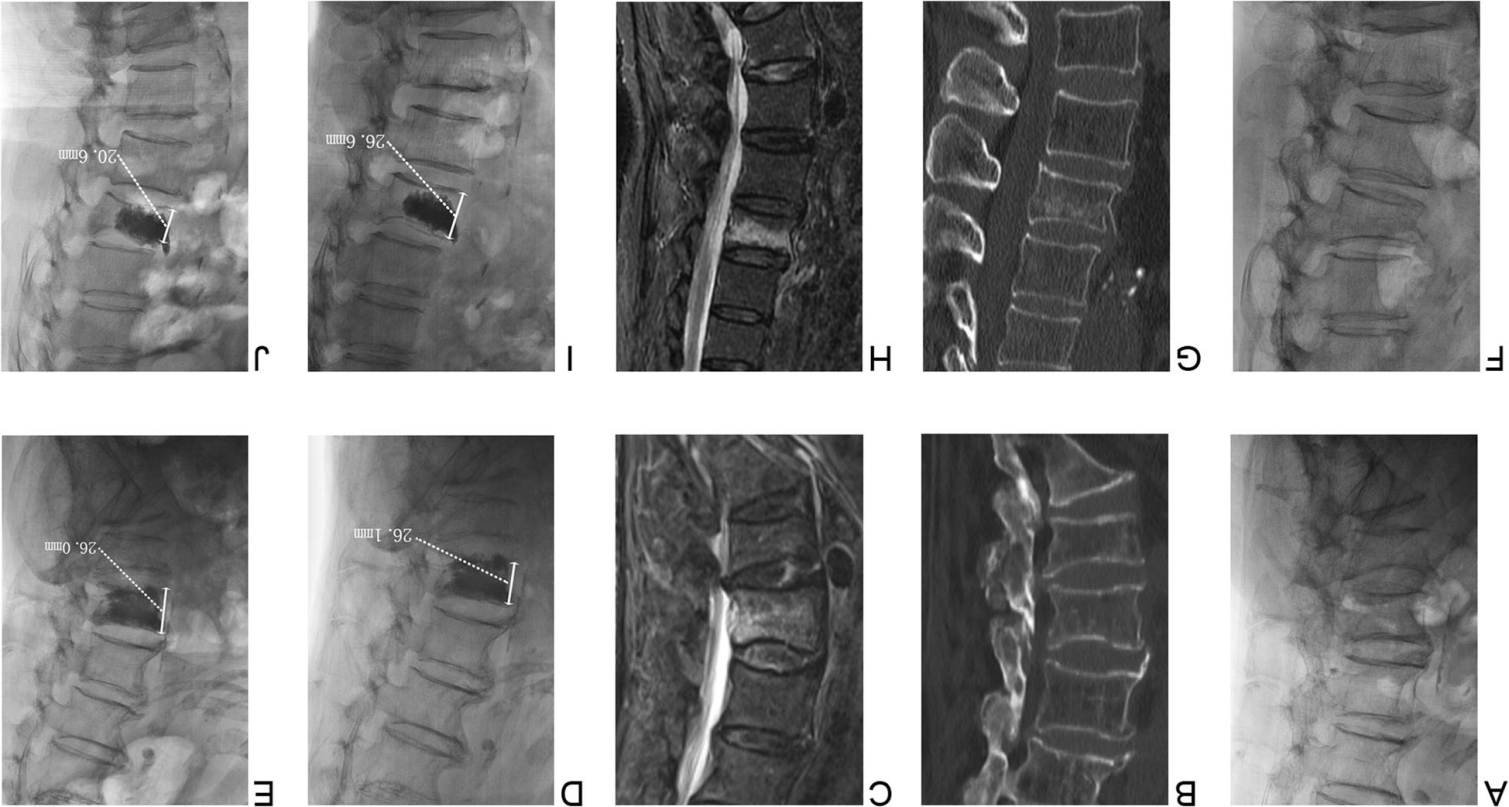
Typical cases. A 71 years old female patient, preoperative x-ray (a), CT (b), MRI (c) showed acute OVCF of L4. X-ray (d) at 24 h post operation showed that the cement was in close contact with the upper and lower endplates, and X-ray (e) at 12 months post operation showed that the vertebral height was maintained well. A 62 years old female patient, preoperative x-ray (f), CT (g), MRI (h) showed acute OVCF of L2. The X-ray (i) at 24 h post operation showed that the cement did not contact the lower endplate. The X-ray (j) at 6 months post operation showed that the height of the vertebral body was lost and the vertebral body was recompressed
